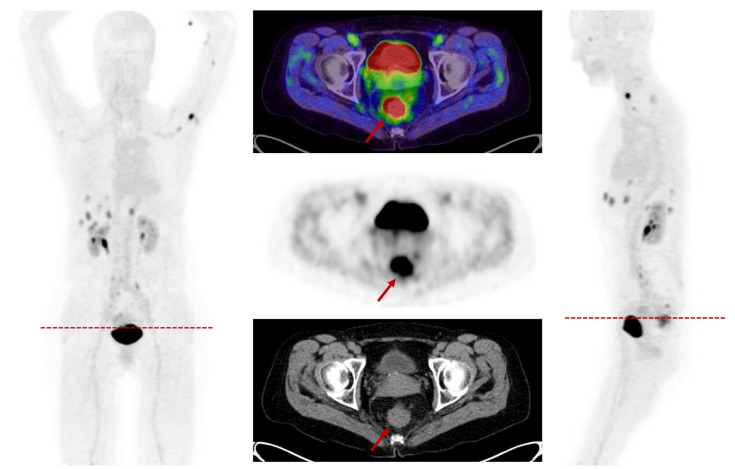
A 57-year-old female patient presenting with newly diagnosed rectal cancer for staging prior to a resection. The FAPI-PET/CT demonstrated a rather strong FAPI accumulation in the rectum with a SUVmax of 15.8. Additionally, the scan showed multiple lymphatic and hepatic metastases.
